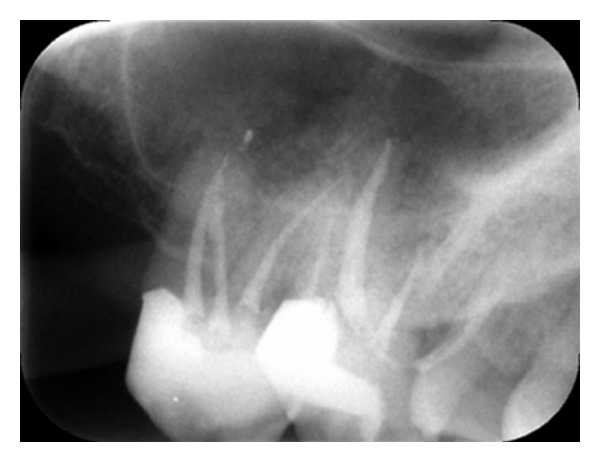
A 1-year follow-up periapical radiograph.
